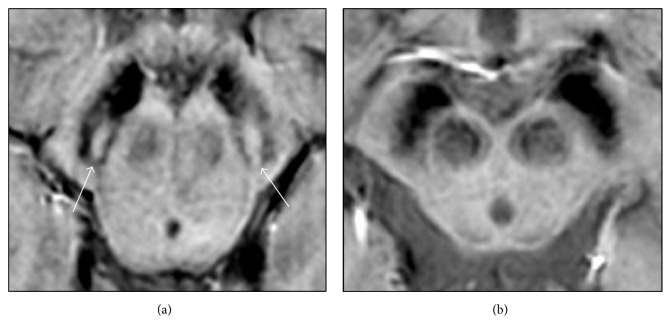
(a) High-resolution MRI (Philips Ingenia 3 T SWIp sequence) in a healthy subject: nigrosome 1 is evident as a linear, comma- or wedge-shaped hyperintense area surrounded by low signal intensity structures of the pars compacta of the substantia nigra, resembling a swallow tail (arrows). (b) In a patient with PD, nigrosome 1 is not visible bilaterally.
